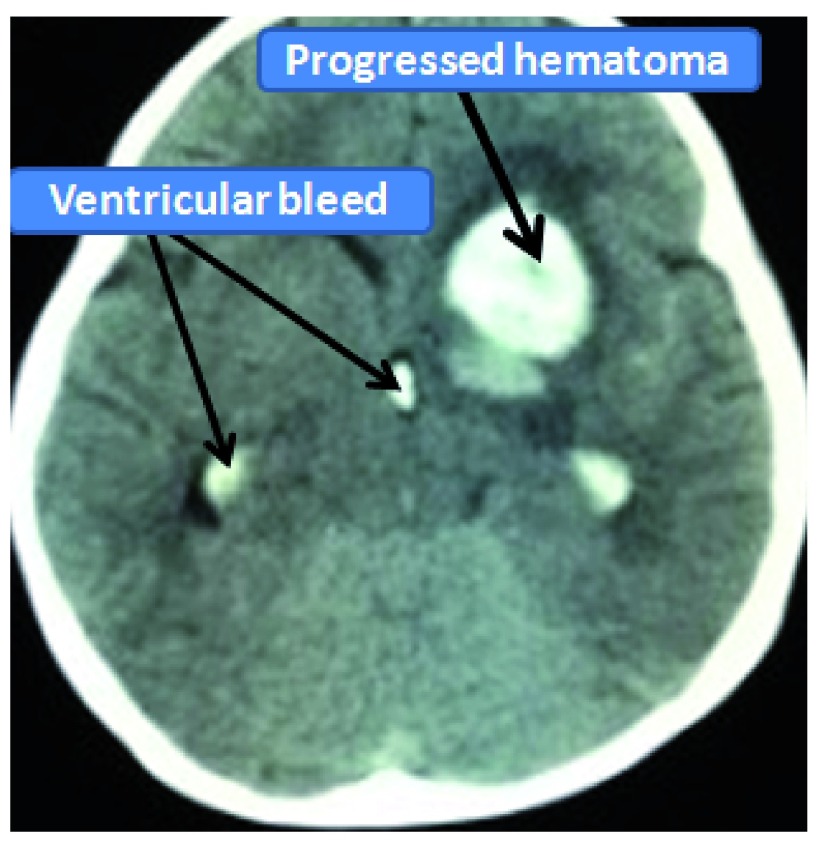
CT head showing evidence of re-bleed in the previous territory along with ventricular extension.
